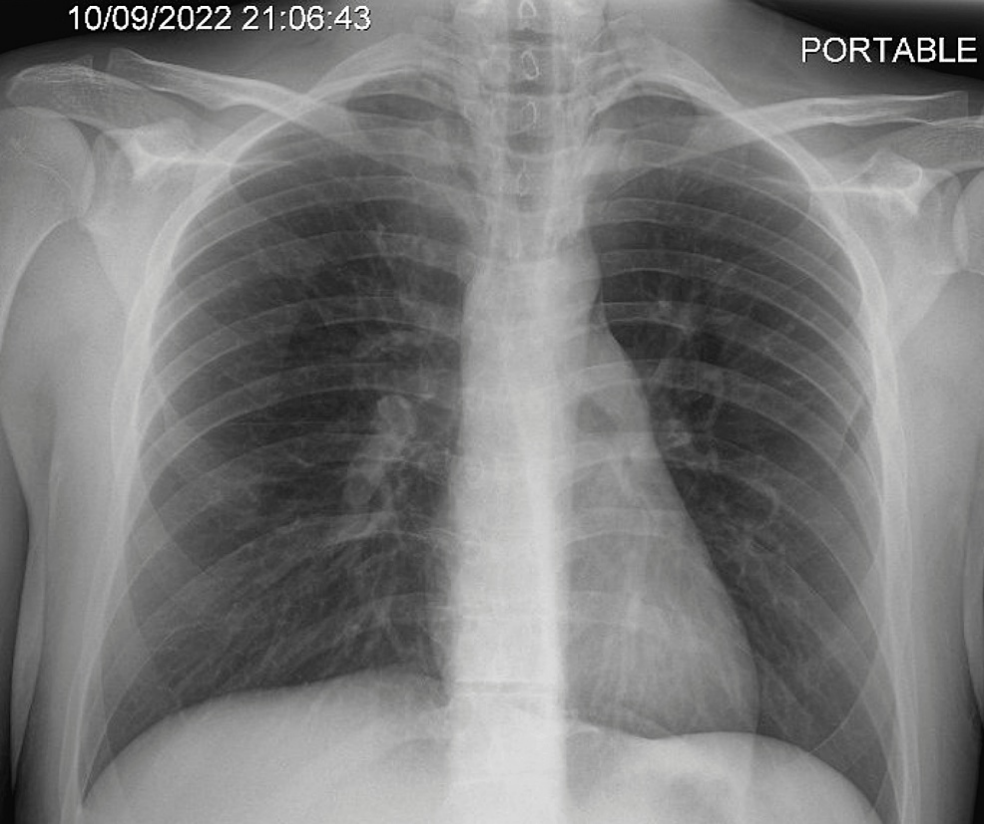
Chest X-ray reveals signs of pneumomediastinum with the presence of air surrounding the heart and in the subcutaneous tissues of the neck.
